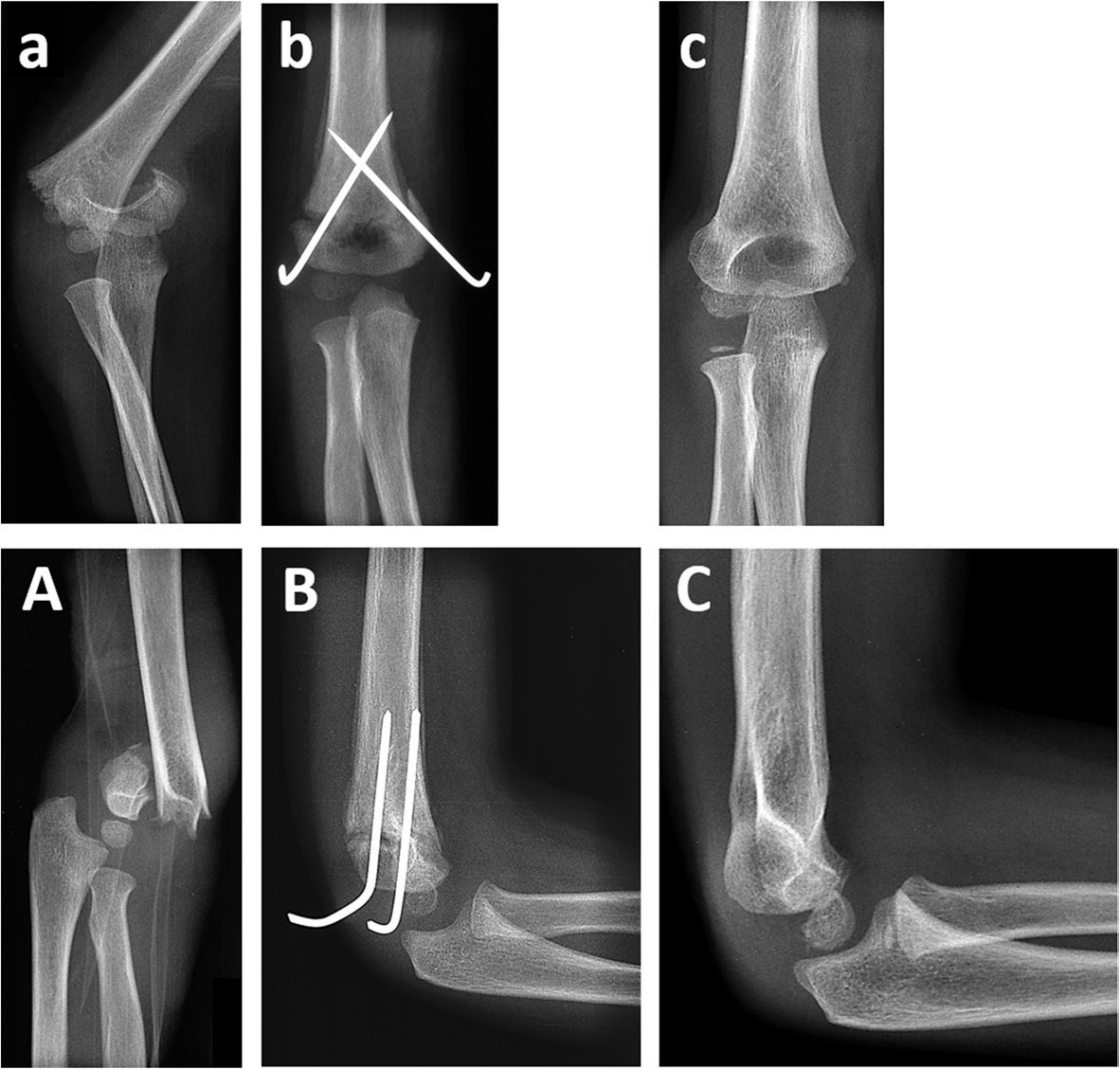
A.p. (a) and lateral (A) elbow radiographs of a displaced SCHF in a five-year old boy, which was treated surgically by closed reduction and crossed K-wire fixation. Consolidation radiographs after three weeks (B,b) showed a small remaining varus deformity on the a.p. view (b) and no rotational deformity laterally (B). At one year follow-up complete remodeling has occurred except for an indentation of the lateral distal humerus contour (C,c)
